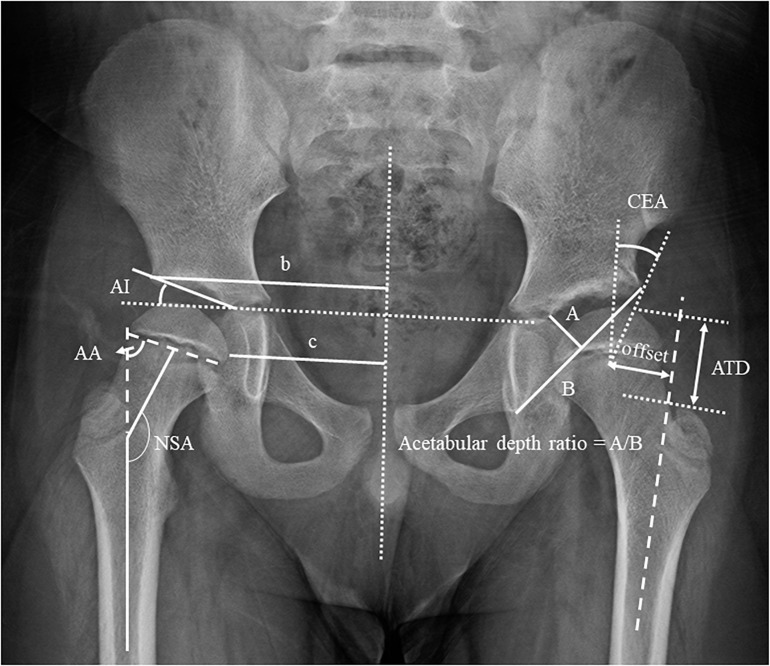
Definitions of the radiographical measurements of the morphology of the hip. NSA, neck-shaft angle; AI, acetabular index; CEA, center-edge angle; A/B, acetabular depth ratio; ATD, articulotrochanteric distance; c/b ratio; AA, Alsberg angle.
